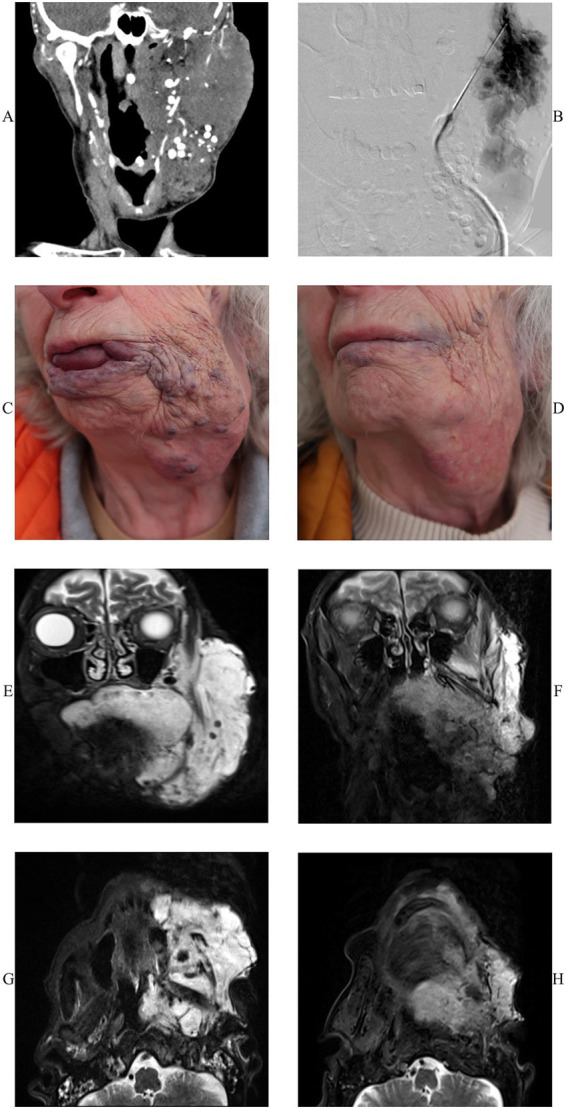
A 78-year-old patient with an extensive venous malformation (VM, indicated by arrows) undergoing two sessions of Bleomycin-Electrosclerotherapy (BEST). (A) Computer-tomographic angiography (CTA) of the patient demonstrating a large mass involving the left cheek with numerous phleboliths. (B) Digital subtraction angiography (DSA) demonstrating the distribution of contrast in one part of the venous malformation (VM). (C) Image of the patient before therapy demonstrating the large VM with involvement of the lips and the tongue. (D) Image of the same patient 8 months after the second session showing a good response with a marked response and without the typical skin discolorations following BEST. (E,G) T2-weighted (T2w) coronal and axial magnetic resonance (MR) images of the same patient before therapy demonstrating the extensive VM. (F,H) T2w coronal and axial images demonstrating a strong reduction of the treated VM.
